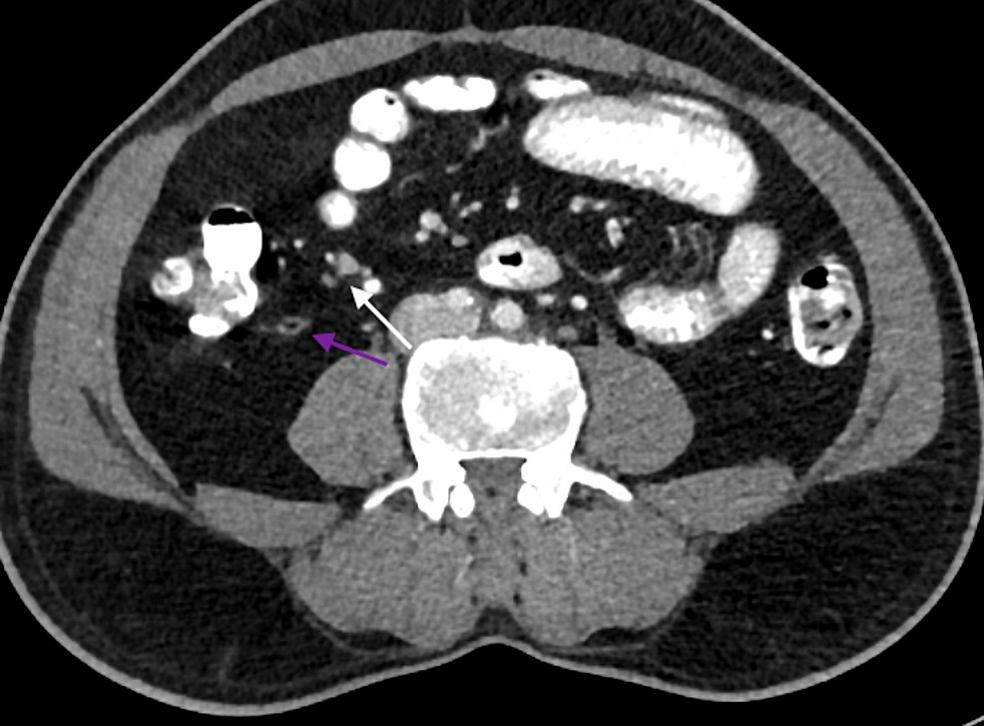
Contrast-enhanced CT abdomen axial view showing normal appendix with intraluminal air (purple arrow). The white arrow shows enlarged mesenteric lymph nodes.
